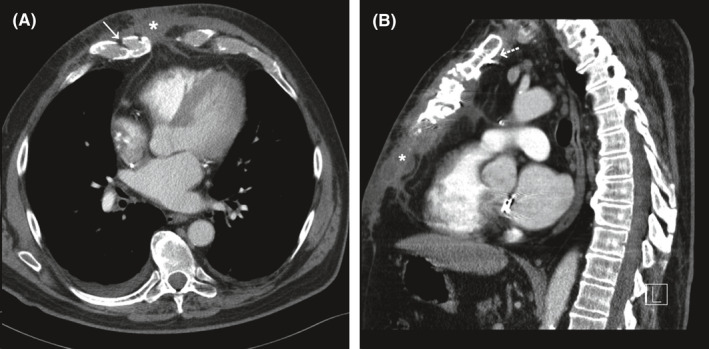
Chest computed tomography axial (A) and sagittal (B) sections demonstrating recurrent infection. White arrow indicates sternal sclerosis. White asterisk indicates substernal fluid collection tracking to the inferior wound. White dashed arrow demonstrates a retained sternal wire
